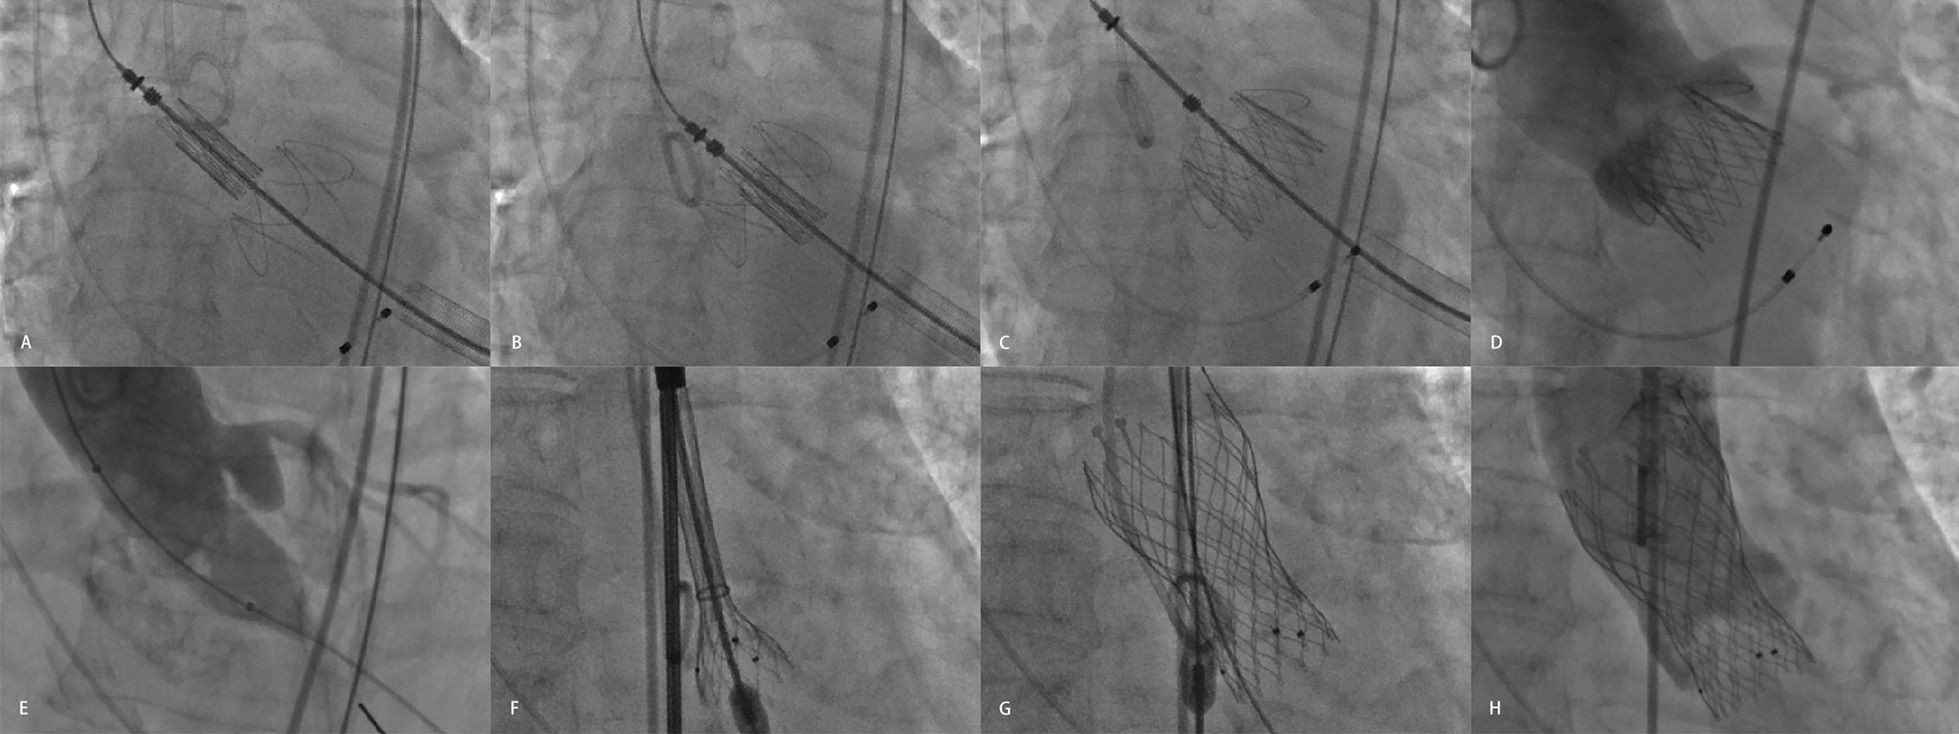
Measurements of the aortic root. A Annulus based on computed tomography (CT) measurements. B Left ventricular outflow tract (LVOT) based on CT measurements. C Sinotubular junction (STJ) based on CT measurements. D Sinus of Valsalva (SOV) based on CT scan. E Annulus based on 3-dimensional transesophageal echocardiography (3D-TEE). (F) LVOT based on 3D-TEE. G STJ based on 3D-TEE. H SOV based on 3D-TEE
